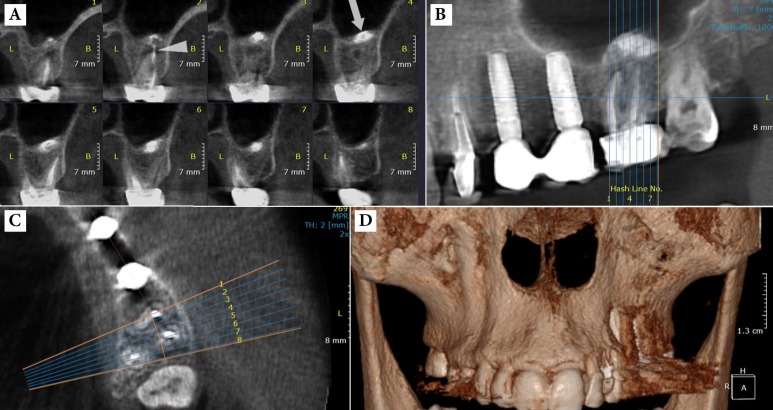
A*) Cross-sectional CBCT view showing the extrusion of sealer after root canal therapy (RCT) of the left maxillary first molar. This image also represents the anatomical relation of roots and maxillary sinus; *B*) Note the extrusion of the sealer through the periapical lesion into the maxillary sinus. *C*) Anatomical relation of roots and buccal/palatal cortical plates *D*) Three-dimensional reconstruction
